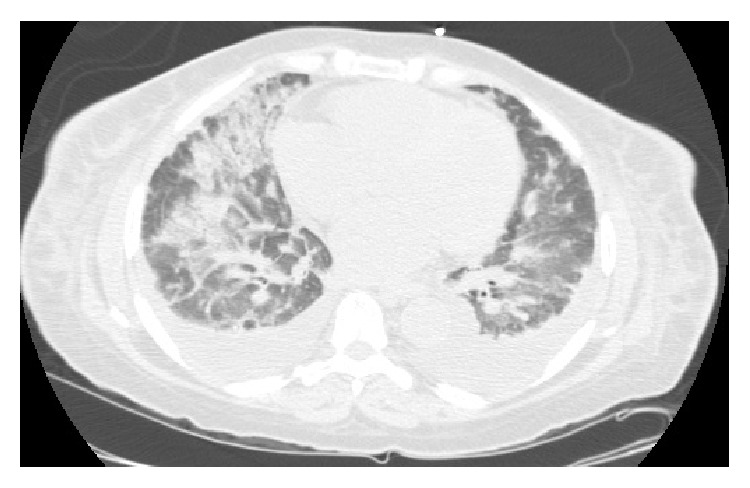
CT chest showing bilateral alveolar infiltrates and ground glass opacities.
